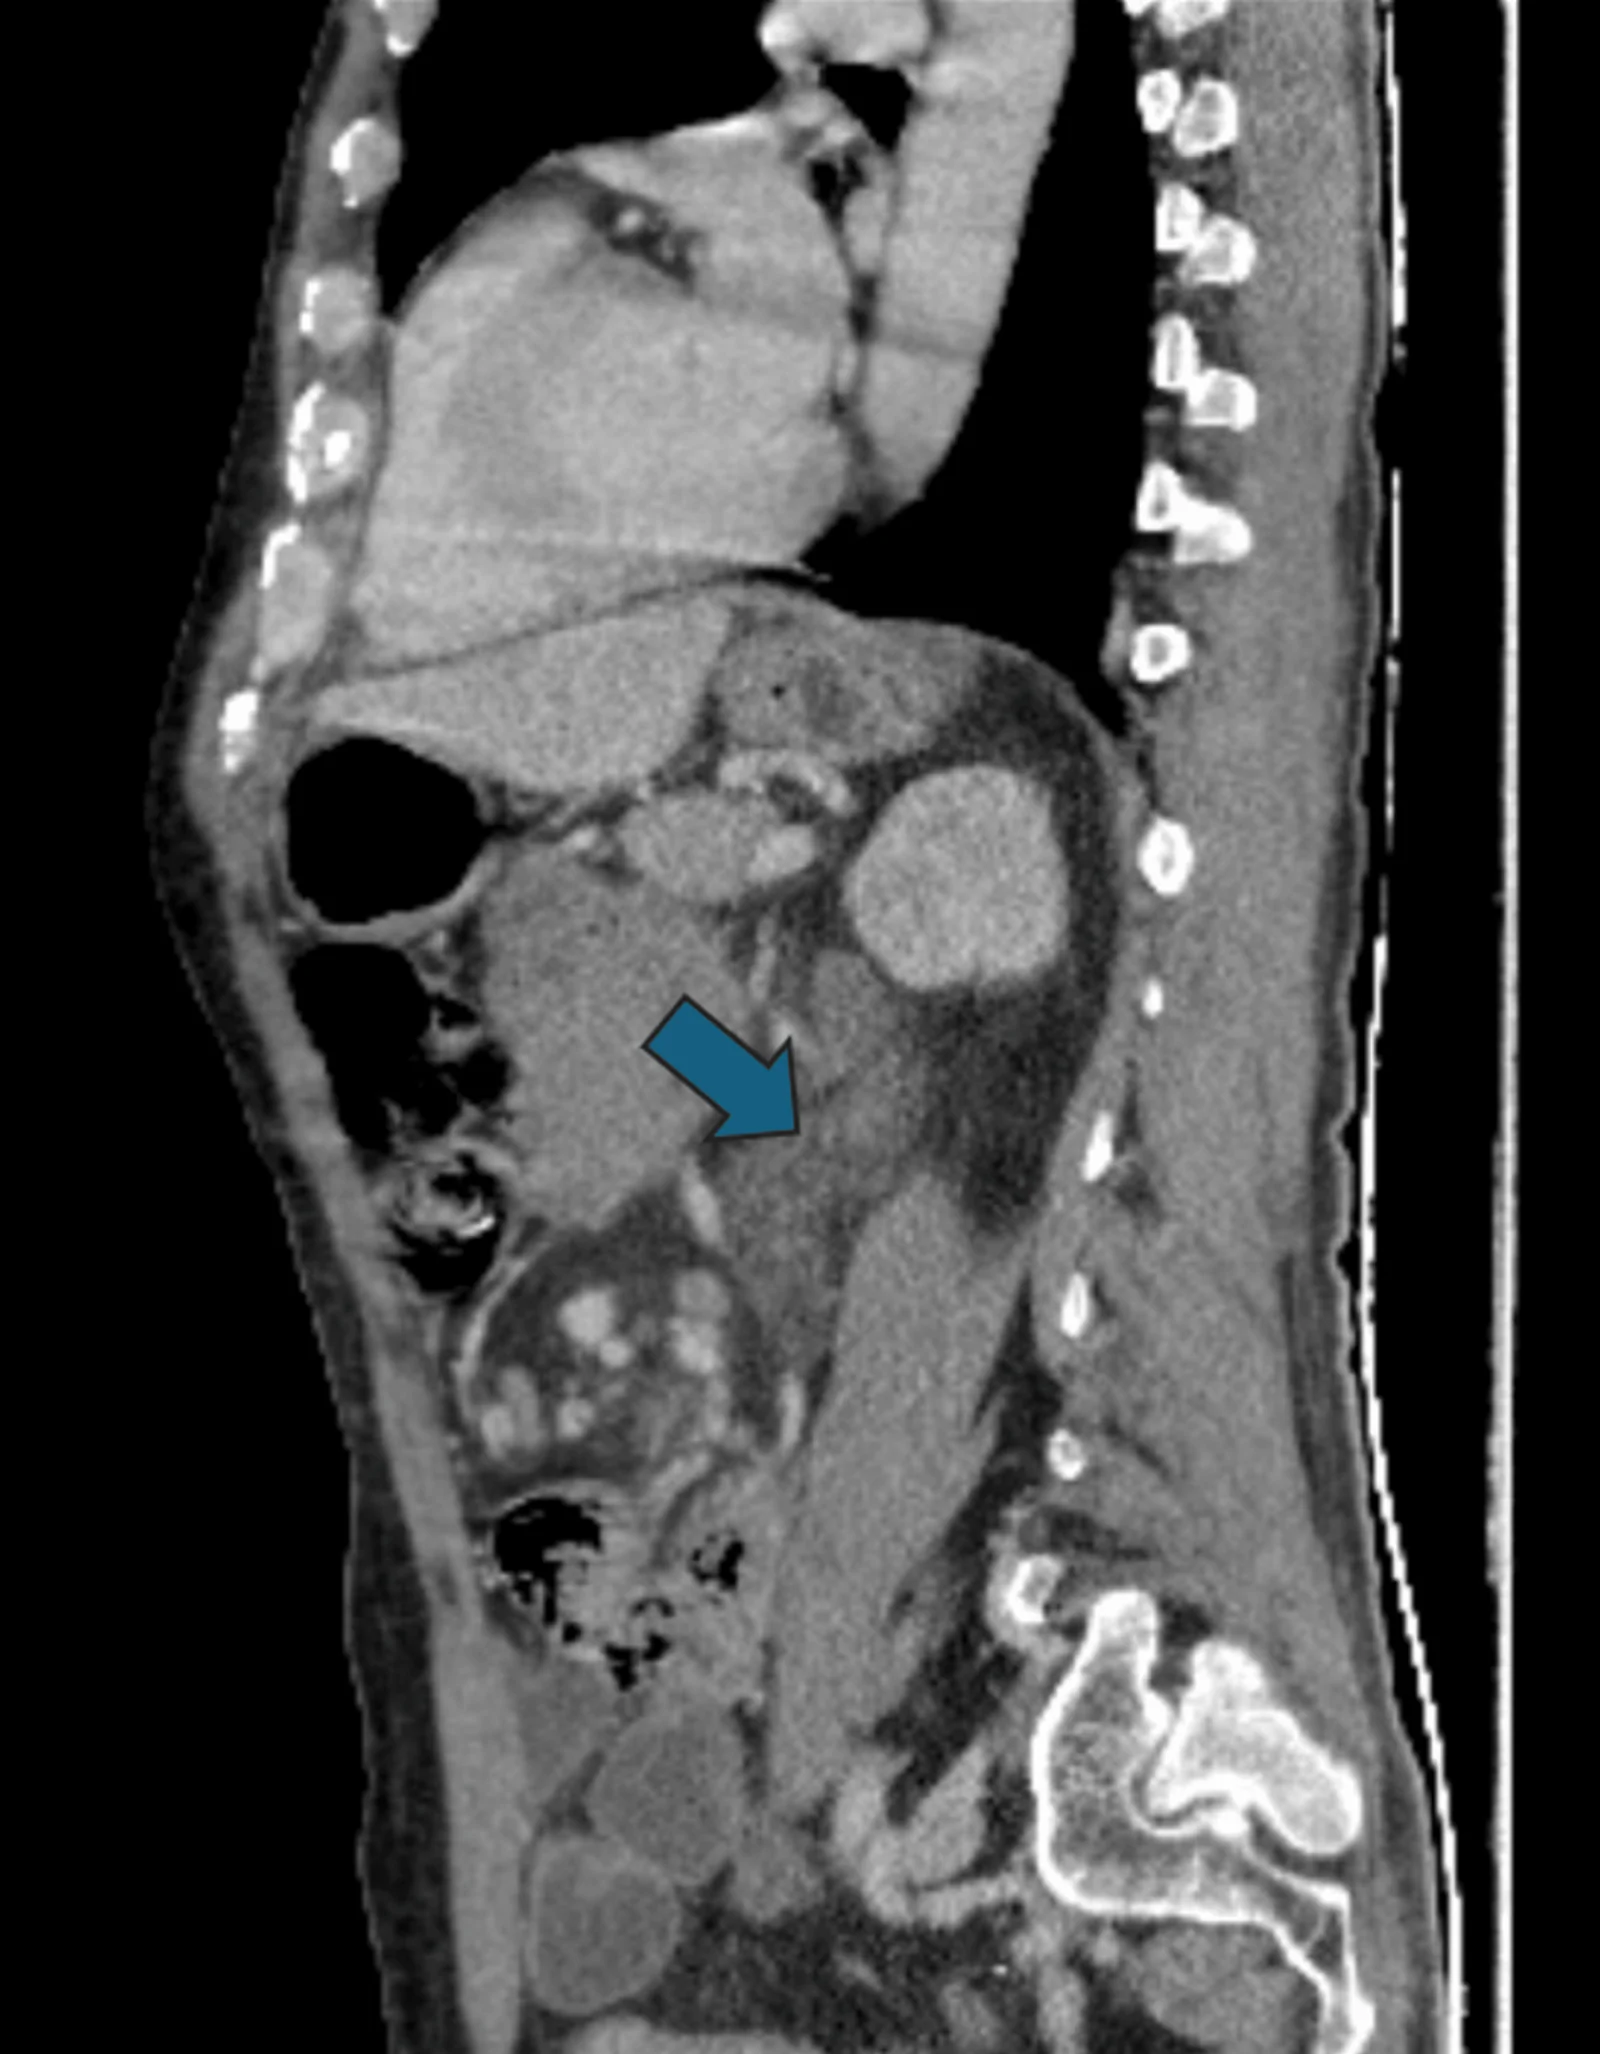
Contrast-enhanced abdominal CT shows bulky retroperitoneal lymphadenopathy (blue arrow)
